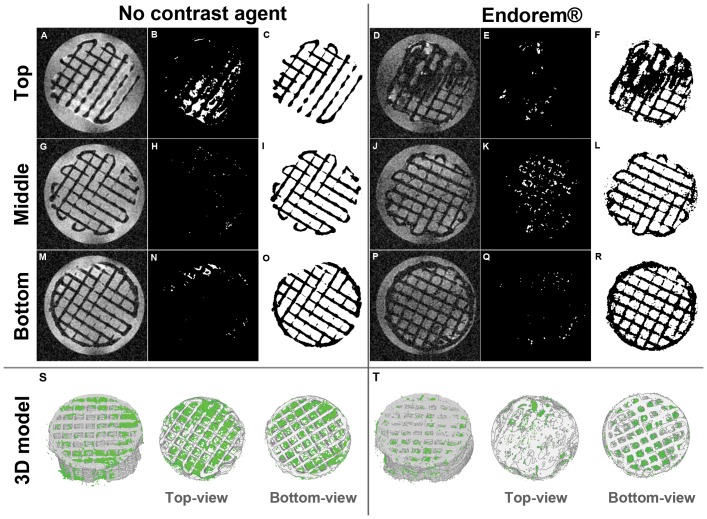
MRI scans presented by a single slice from each of the following volumes; top, middle and bottom of the scaffold after 5 weeks of culture (donor 2) obtained without the use of any contrast agents (A, G and M) and after the addition of Endorem T_2_ contrast agent (D, J and P). Tissue is identified and appears white after image processing (B, H, N, E, K and Q). Scaffold material is extracted from unprocessed images by image processing sequences (C, I, O, F, L and R). By a mathematical combination of the images representing tissue-material and the images displaying scaffold material, a 3D model could be retrieved (S and T).
